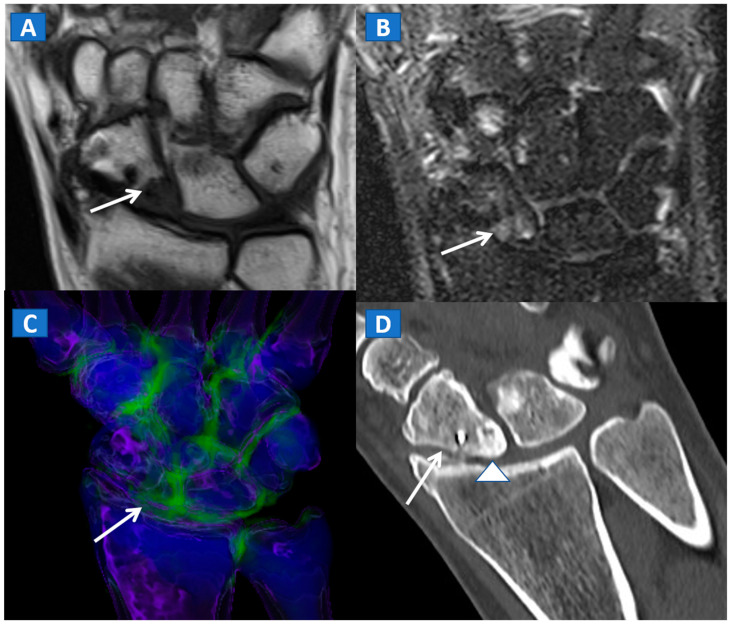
A 45-year-old male with persistent pain after surgical fixation of a middle-third carpal scaphoid fracture. On the coronal T1-weighted (A) and STIR (B) MRI images, metal-induced and motion artifacts impede the correct evaluation of the healing process. Furthermore, subtle BME is depicted proximally (arrow). On the 3D DECT image (C), some proximal BME can be confirmed (arrow). On the 1 mm reconstructed standard CT image on the coronal plane (D), the BME appears as an area of increased density (arrowhead). There are no signs of any residual fractures adjacent to the inserted metallic hardware.
